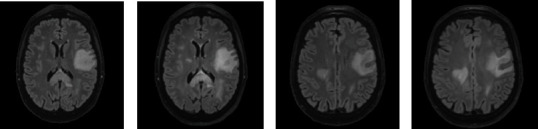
Increased FLAIR hyperintensity prior to (a, c) and 6 days after starting methylprednisolone (b, d). A new FLAIR hyperintense lesion was seen laterally to the right lateral ventricle (b).
